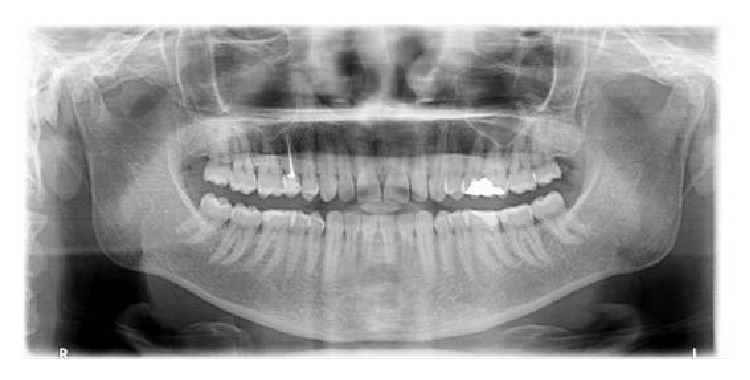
Radiographical image of patient.
